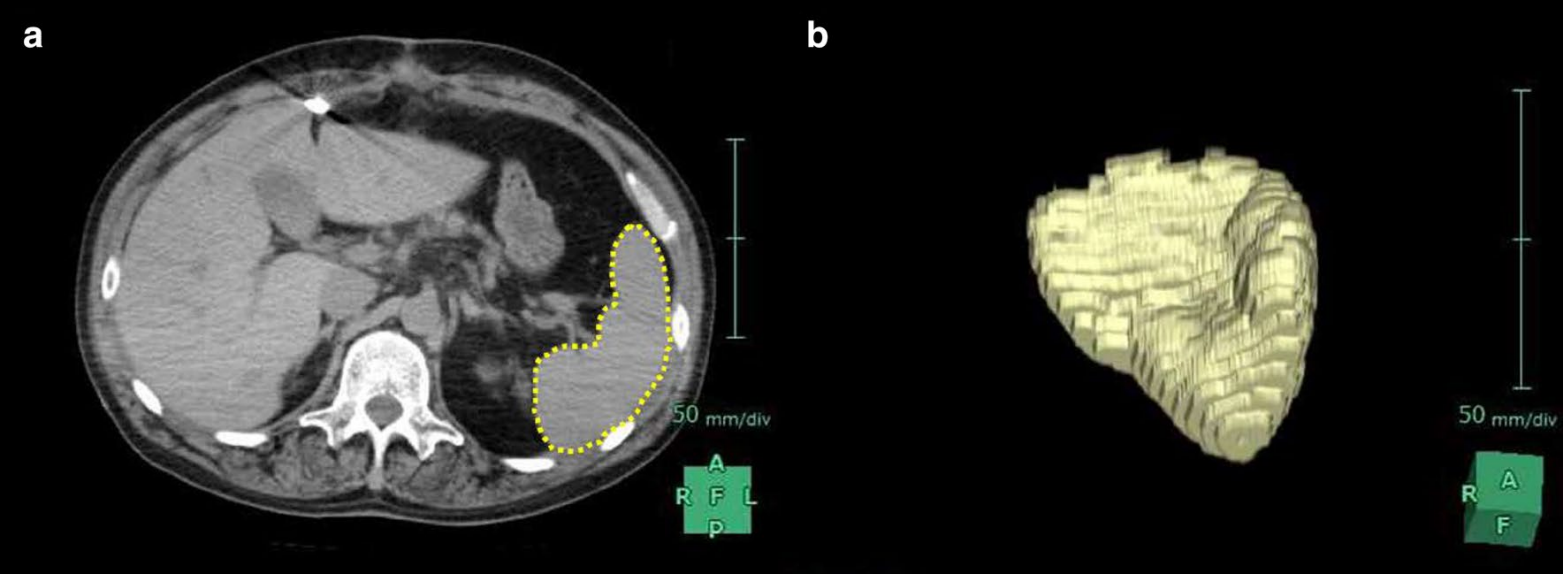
Abdominal computed tomography axial-view image from a patient with a left ventricular assist device. The spleen is surrounded by a yellow dotted line (a). Three-dimensional images were obtained by outlining the spleen (b)
